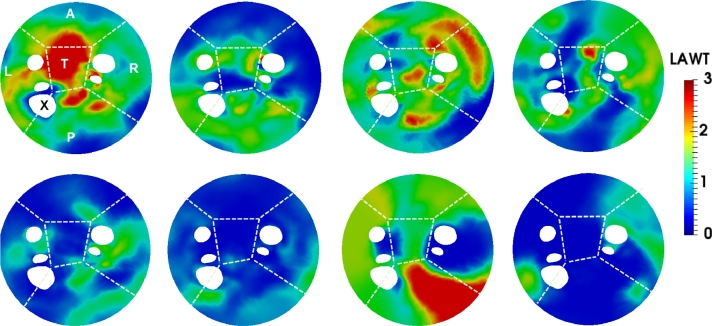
Flat 2D compact representations of thickness maps of eight images from the CT database to allow standardised comparison. In each flat map, the five holes represent four pulmonary veins and the left atrial appendage marked with an X. Each map is divided into separate sections as indicated by labels L (left), R (right), A (anterior), P (posterior), T (roof).
